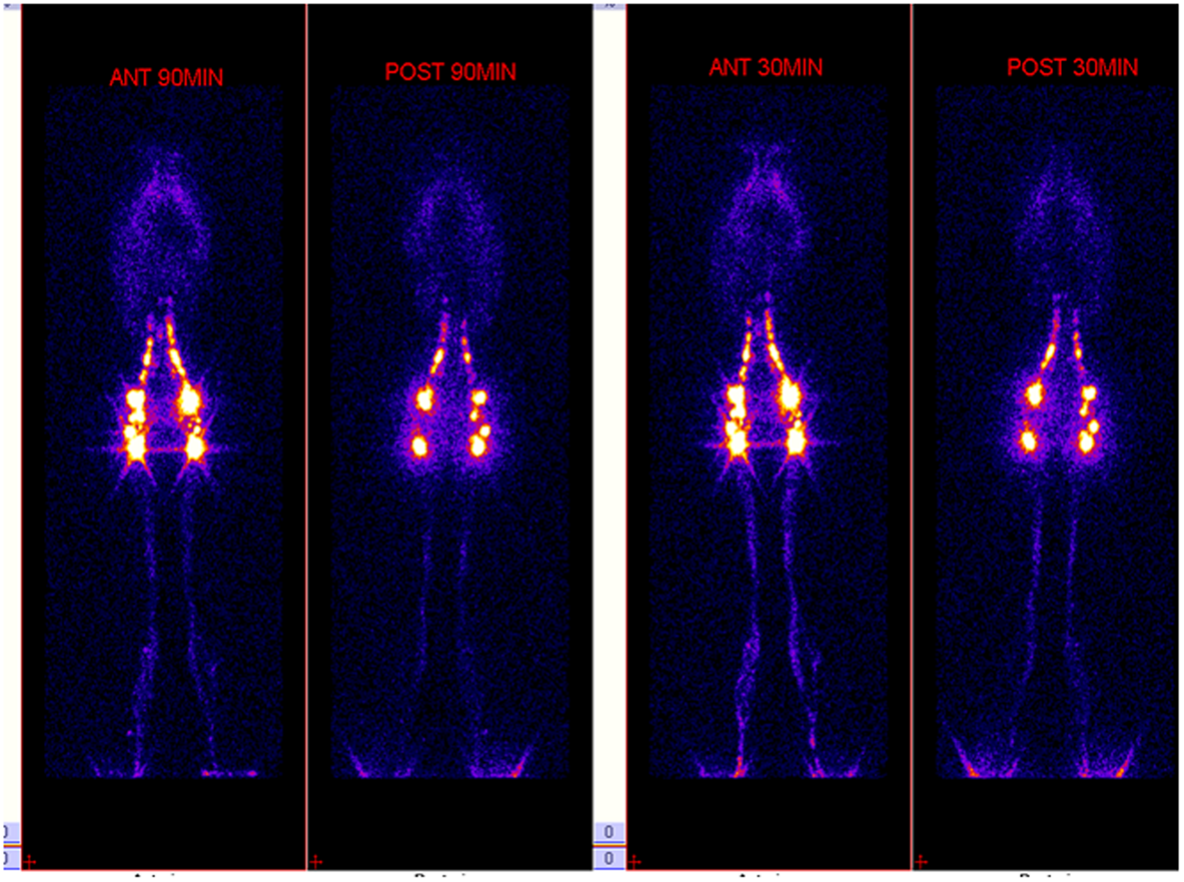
A Lymphangioscintigraphy (radionuclide lymphangiogram) of the chest images shows significant radioactive uptake in the chest, which was higher than the liver shadow. Lower limb lymphatic reflux is observed without signs of obstruction
